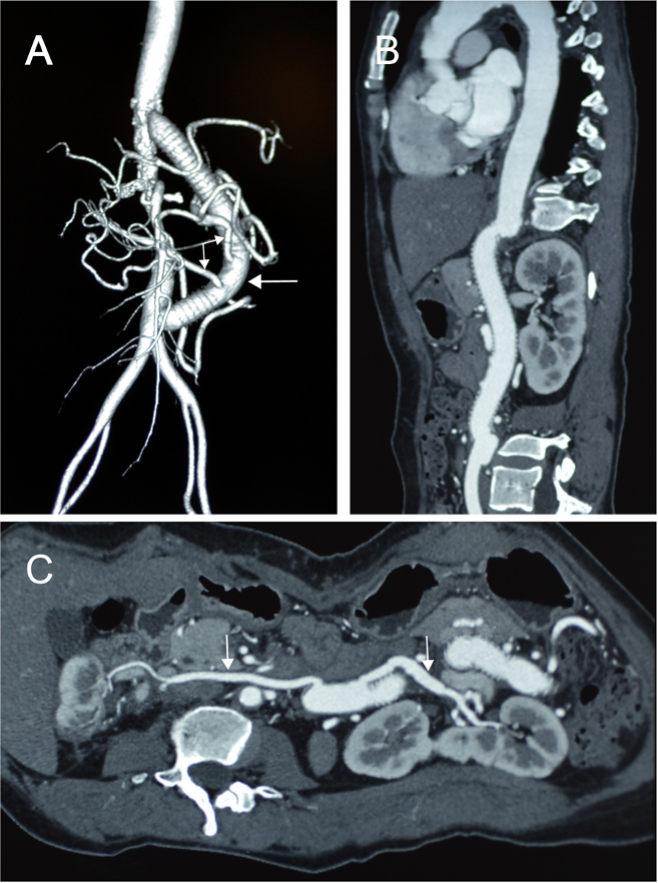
Postoperative imaging showed that all the grafts were patent. A, Postoperative three-dimensional computed tomography angiography showing the extra-anatomic bypass (right arrow) with the autogenous saphenous vein grafts (left arrows). B, Multi-planar reconstruction showing that the new prosthetic graft is patent. C, Curved-planar reformation of the computed tomography angiography showing that the saphenous vein grafts (two arrows) are patent.
